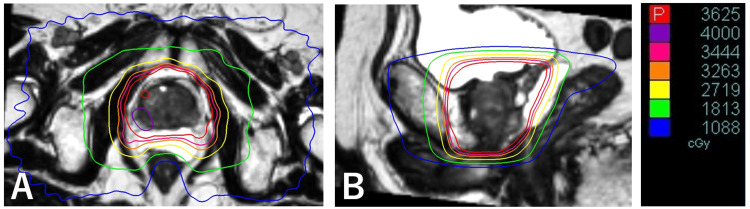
Dose distribution of prostate SBRT The prostate SBRT treatment plan is displayed on fused T2-weighted MRI on the representative (A) axial and (B) sagittal views. Abbreviations: SBRT, stereotactic body radiation therapy; MRI, magnetic resonance imaging
